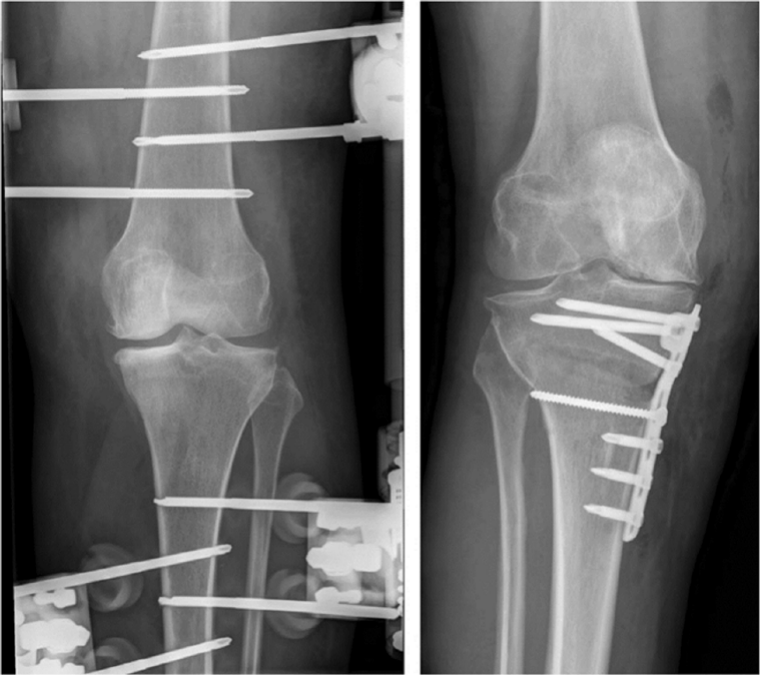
Posteroanterior radiographs of knee joint distraction (left) and high
